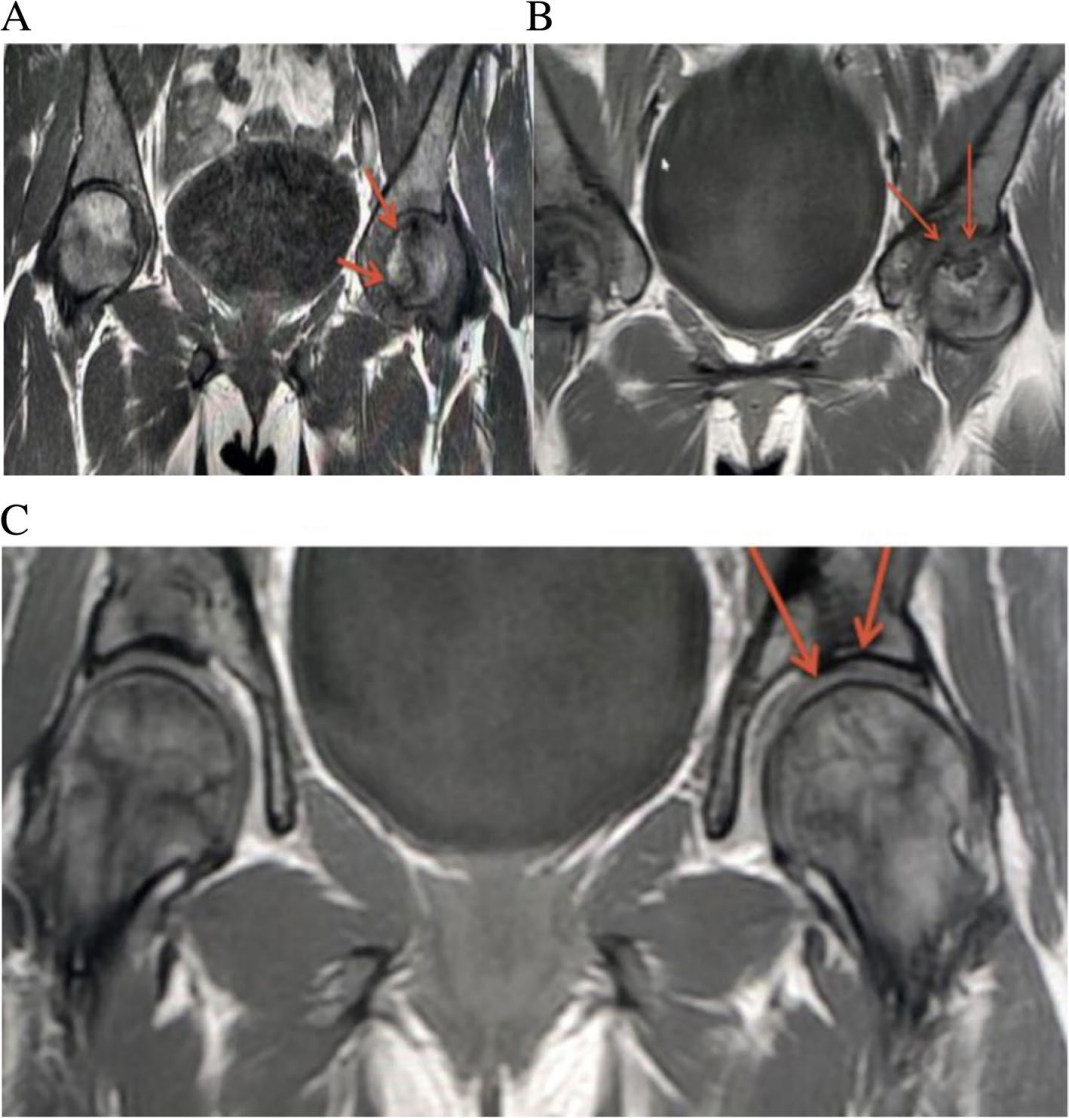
A Coronal T1 weighted image of the pelvis demonstrates a large geographic area of ANFH in the anterior-superior aspect of the left femoral head . There is no significant subchondral collapse. B Coronal T1 weighted image perfomed 4 months after demonstrates interval improvement of femoral head ANFH. C MRI done after 30 months. Coronal Short-TI Inversion Recovery image demonstrates near complete resolution of the femoral head ANFH
